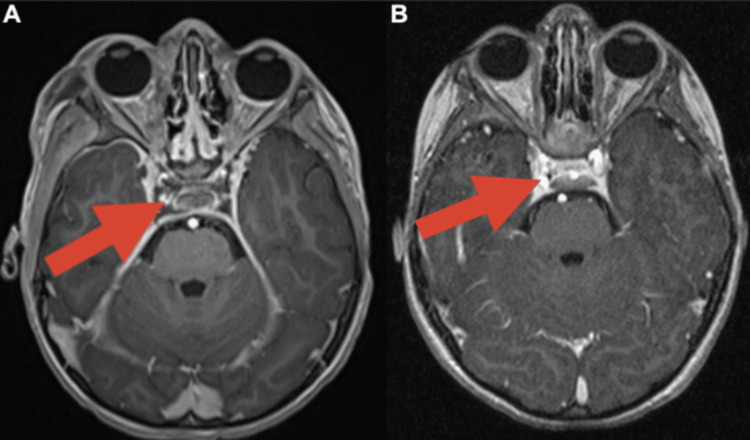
MRI comparison of a cavernous sinus thrombosis patient and a healthy patient. A: T1 post-contrast (magnetization-prepared rapid gradient-echo, MPRAGE) axial MRI at the level of the cavernous sinus demonstrates non-opacification of the cavernous sinus following the administration of intravenous contrast. B: For comparison, in a healthy patient, there is contrast opacification demonstrated in the cavernous sinus on a similar sequence post-contrast MRI.
